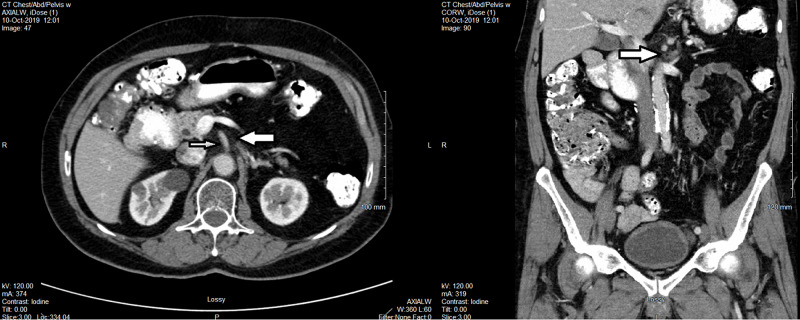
CT scan in October 2019 showing CR. Note the disappearance of residual mass (big arrow) beside SMA (small arrow). CR, complete response; SMA, superior mesentery artery
